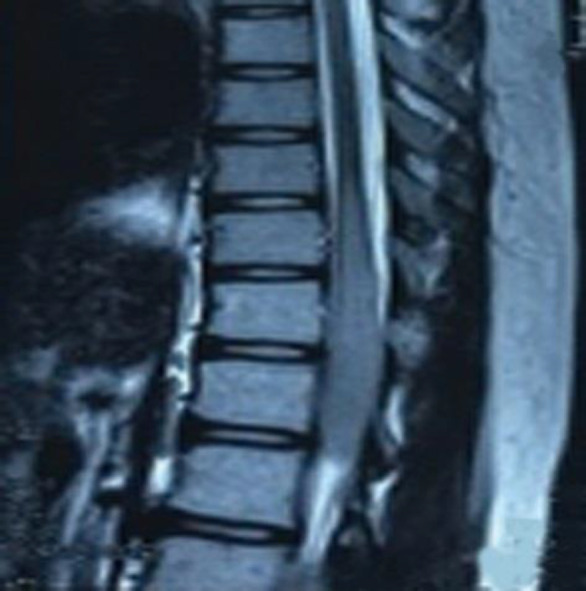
Thoracolumbar MRI revealed ill-defined intramedullary lesion
